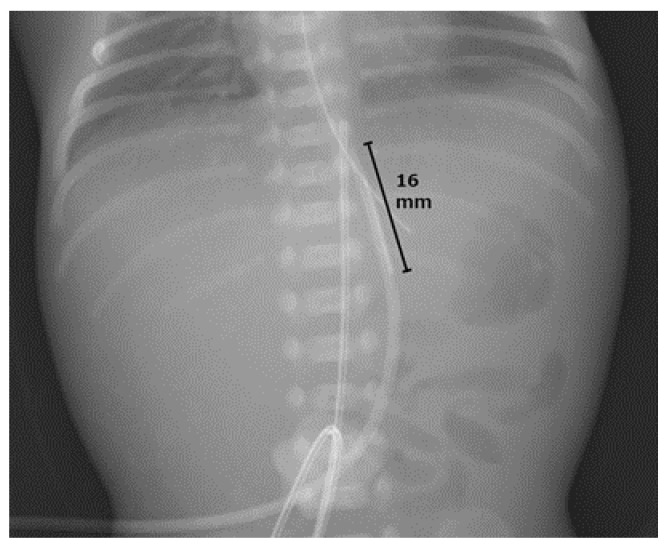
Frontal anteroposterior X-ray of the abdomen just after umbilical vein catheter placement on the day of birth. It shows the tip of the umbilical venous catheter at the Th10 level and the proximal port located 16 mm from the tip.
